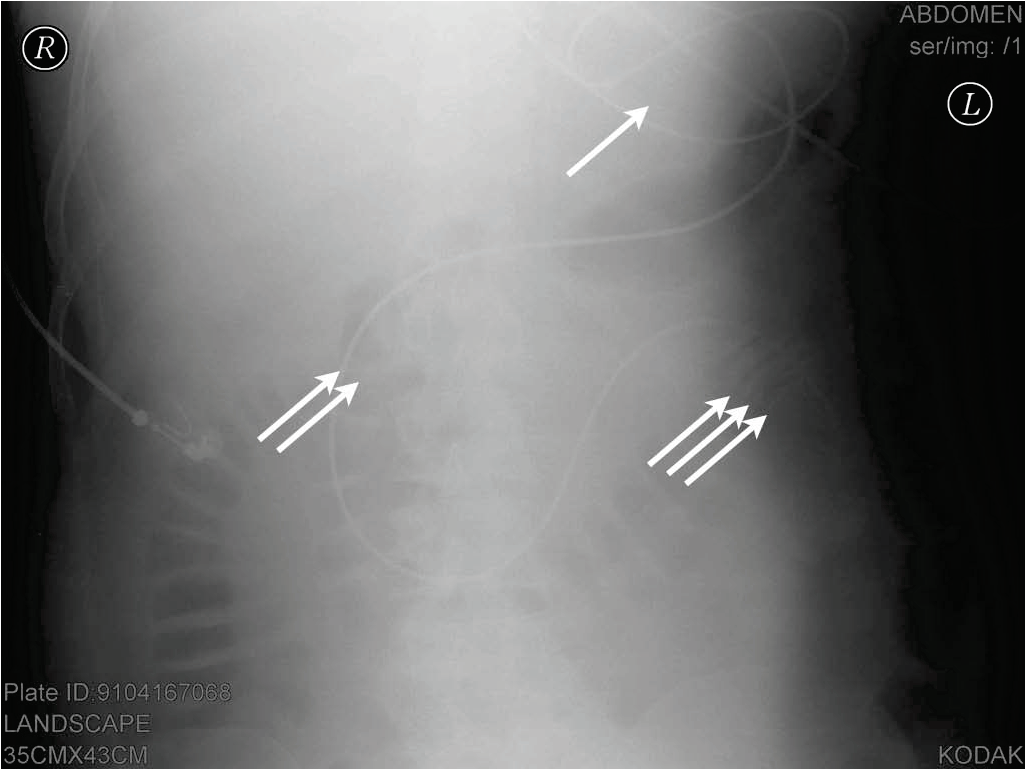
Abdominal X-ray showing the position of the feeding tube. Abdominal X-ray examination after the placement of the feeding tube in patient 8. Loop formation in the stomach (one arrow), the location of the tube in the duodenum (two arrows) and the contrast medium lining the jejunal wall (three arrows) are indicated.
